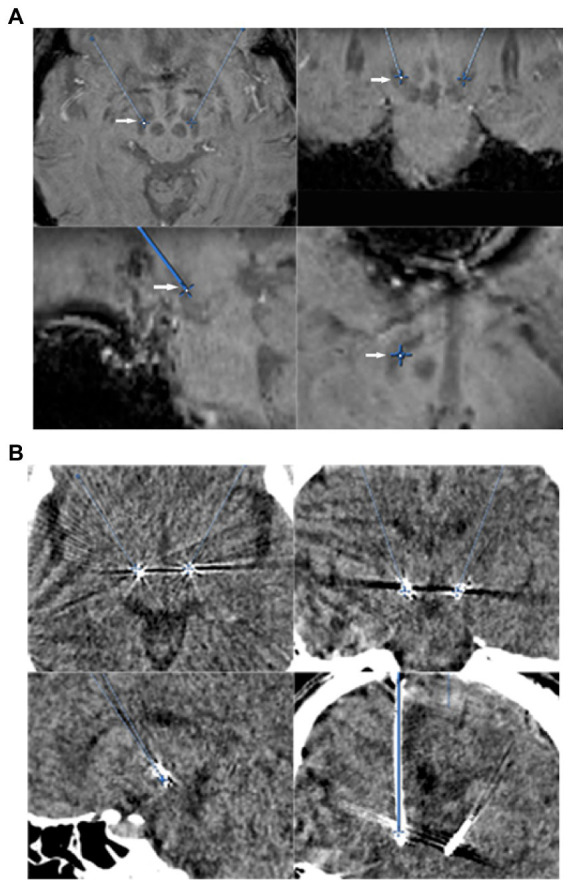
(A) STN direct targeting (arrows) on an SWI-sequence. Axial (upper left), coronal (upper right), and sagittal (lower left), trajectory view (lower right). (B) Post-electrode placement CT scans showing contact place to target, axial (upper left), coronal (upper right), and sagittal (lower left), and trajectory view (lower right).
